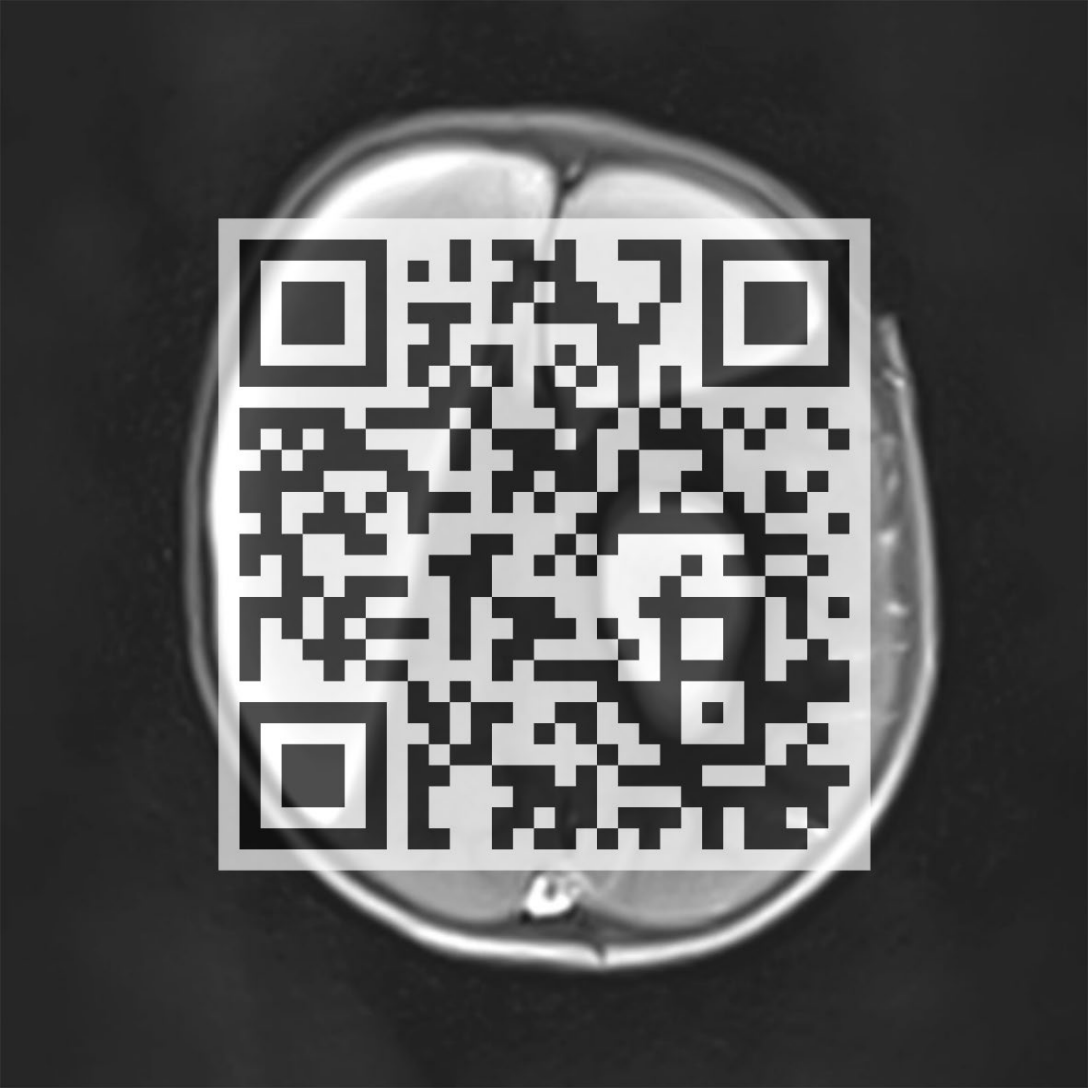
Video 2 Brain volume coverage (T2/T1 weighted) of a 3-year-old boy with a malformation in the frontal brain. The anterior horns of the lateral ventricles are not covered by parenchyma until they reach the rostral skull. A subdural hygroma has developed on the right side. On the left side, a narrow membrane separates the enlarged left lateral ventricle from the external subarachnoid space (red asterisk). Additionally, there is a shunt catheter with a tip in the right subarachnoid space. This patient underwent a total of 21 brain MRIs due to relapsing shunt dysfunction. 18 of the examinations have been performed without anesthesia by real-time MRI, only by gentle fixation of the child by a parent
